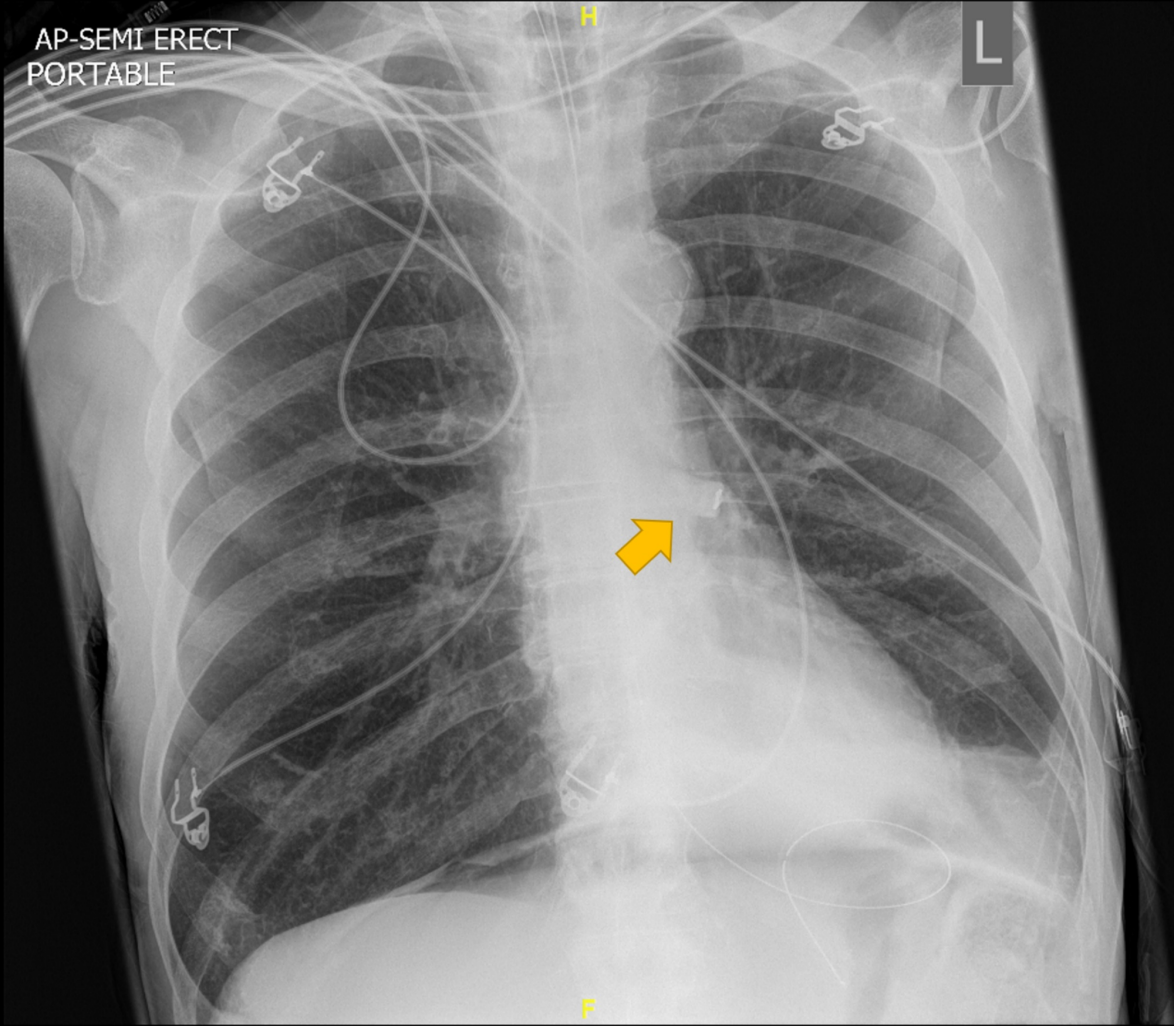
Radiograph showing a tooth in the left main bronchus.
